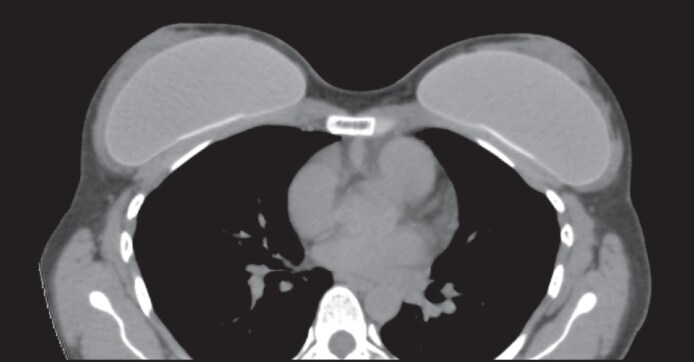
Tomography of breast in a 37-year-old female, 5 months after an ultrasonic breast implant. The breast gland can be seen above and lateral to the implant.
